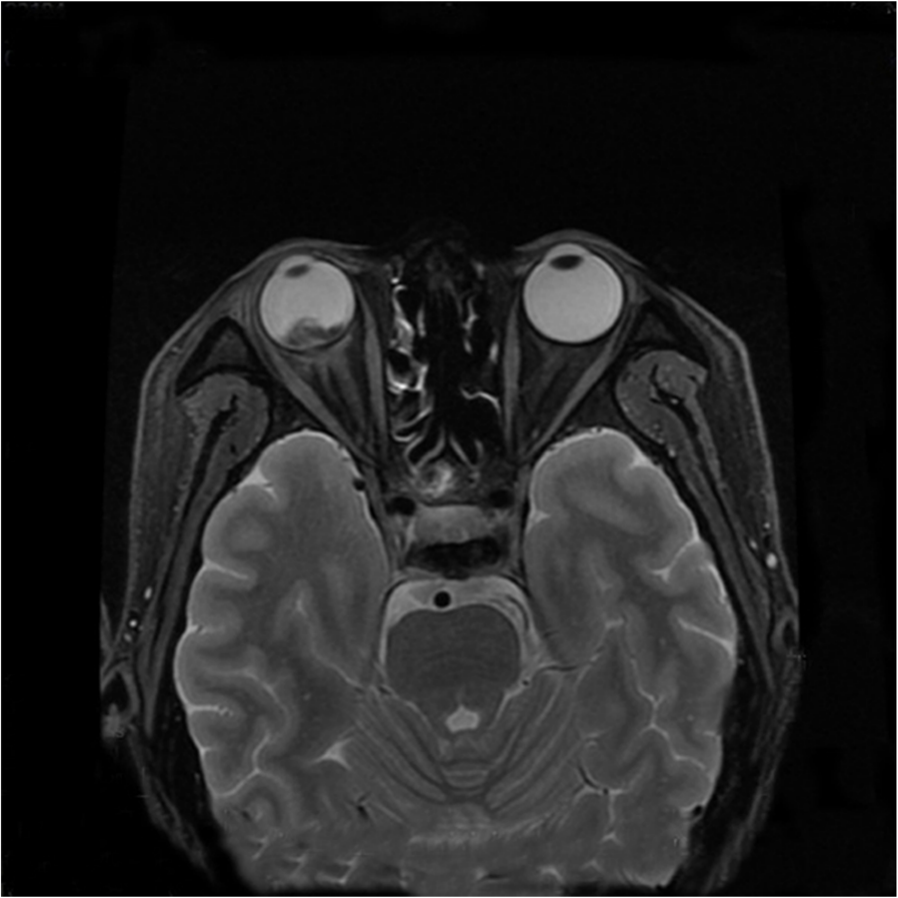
Orbital magnetic resonance imaging revealed fat suppression on T2-weighted images. The right ocular was proptosis. The strip-like hypointense in the posterior wall of the eyeball and flaky hyperintense in the intramuscular fat gap was observed
